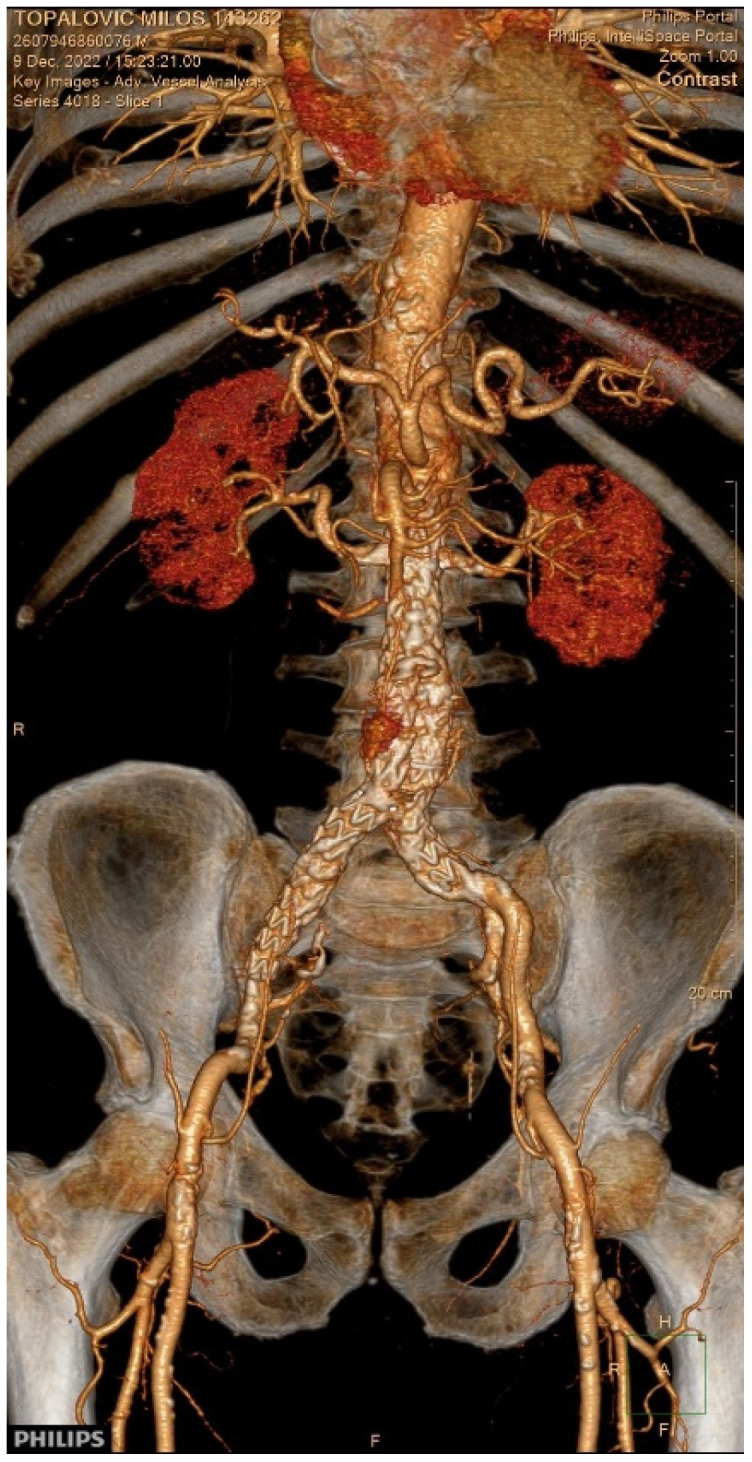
Three-dimensional computed tomography reconstruction of EVAR demonstrating a type II endoleak from the lumbar artery.
